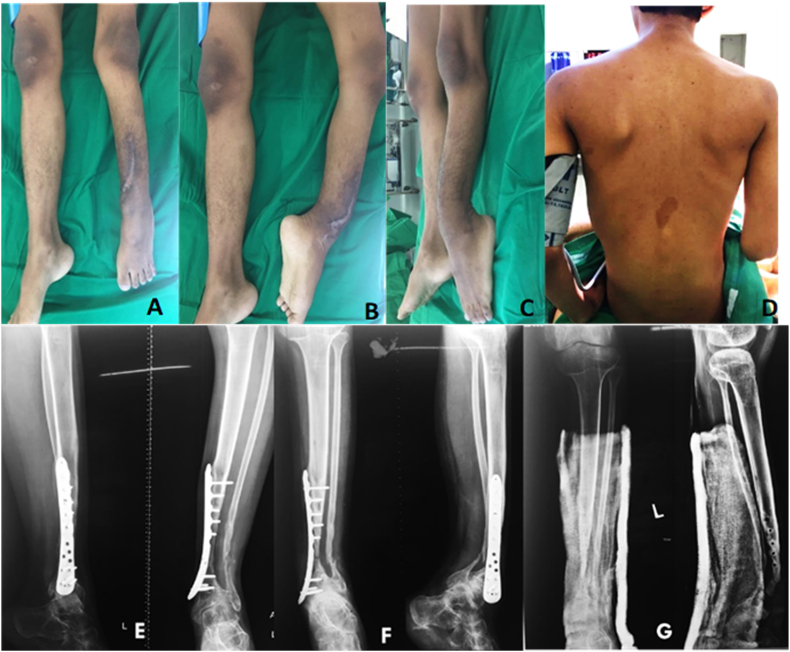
Clinical picture at first admission. A-C Antero-lateral angulation and shortening. No signs of infection from the previous scar and surroundings. D. Café au lait spots on patient's back. E. Third revision surgery. F. Failure 8 months after third revision: Loosening of the distal screws and anterolateral angulation with bone discontinuity. G. Plain radiograph after implant removal: Tapering of both fracture ends.
